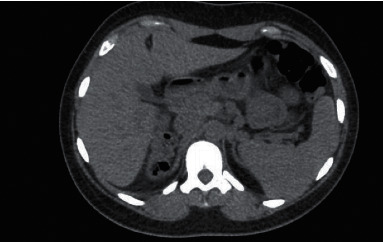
The uncinate process, head and body appear normal in size, but pancreatic tail is not visualized on noncontrast CT scan.
